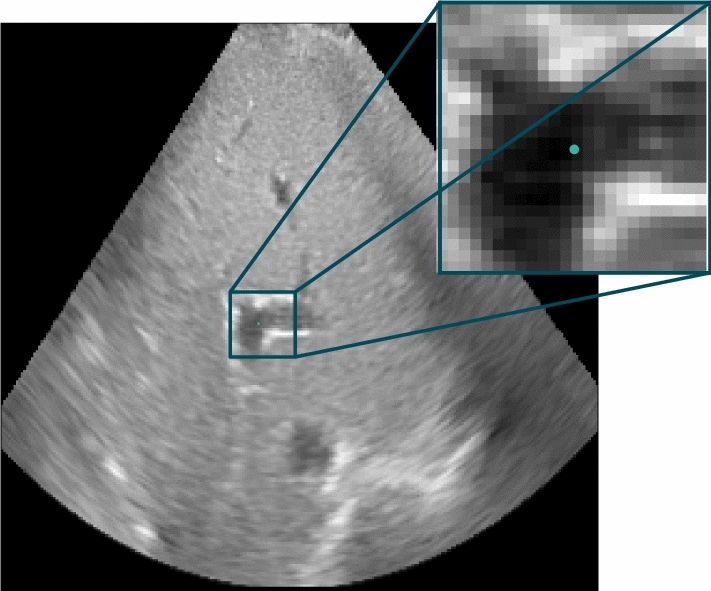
2D slices of an 3D ultrasound volume (left) and a 3D patch (right) selected at the position of an annotated landmark in the data set of subject $$S_1$$
